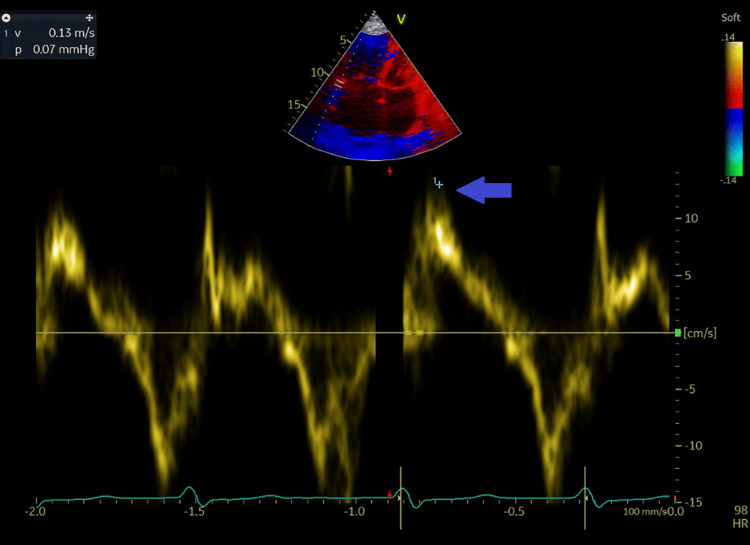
Tricuspid annulus velocity at lateral level by tissue pulsed wave Doppler from the apical window showing normal right ventricular systolic function with an S' at 13 cm/second (blue arrow).
